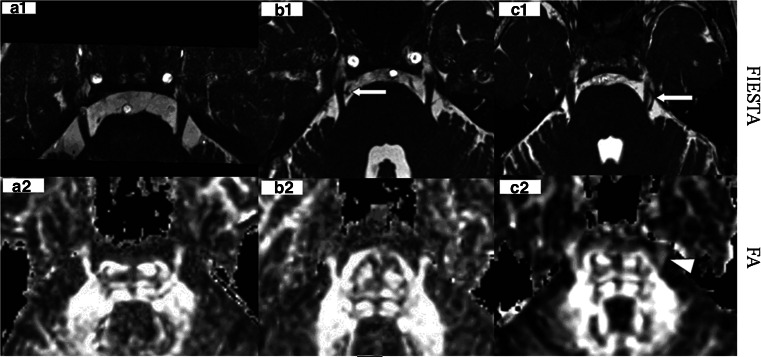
a1 3D FIESTA MR image showing a 53-year-old man (HC without NVC). a2 fractional anisotropy image showing same signal in both *left* and *right* trigeminal nerve; b1 an artery branch (*arrow*) (DCA: 0.70 mm) compressing the *right* trigeminal nerve root in a 52-year-old asymptomatic woman (NVC without TN). b2 fractional anisotropy image showing same signal in both *left* and *right* trigeminal nerves; c1 the proximal SCA (*arrow*) (DCA: 1.56 mm) compressing the *left* trigeminal nerve root in a 58-year-old TN woman (NVC with TN). c2 fractional anisotropy image showing hypointensity signal in the *left* nerve (*arrowhead*) compared with the opposite side. Note: *HC* healthy controls, *NVC* neurovascular compression, *DCA* diameter of compression arteries, *TN* trigeminal neuralgia, *SCA* superior cerebellar artery
